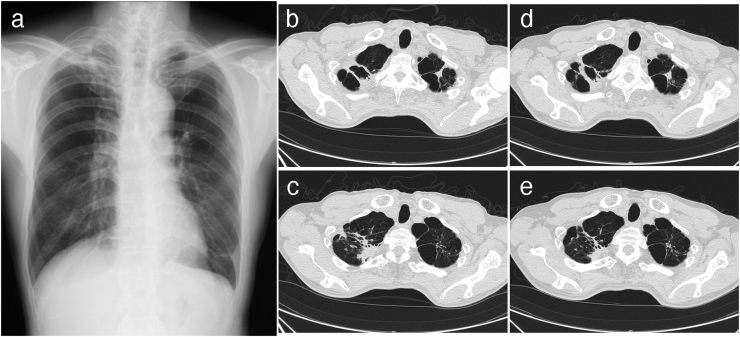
Images of chest X-ray and computed tomography (CT) scan. (a) Chest X-ray at the initial visit shows patchy opacifications with fibrotic shadows in the upper right lung. (b and c) Chest CT scan taken at the initial visit shows fibrocavitary lesions surrounded by emphysematous changes on the right side predominantly in the bilateral upper lobes. These lesions remained stable after 2 years of follow-up (d and e).
